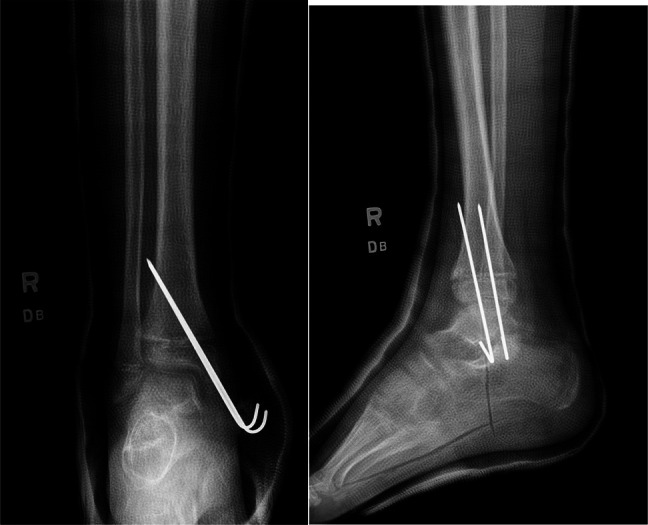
Radiographs showing AP and lateral views of percutaneous pinning.
